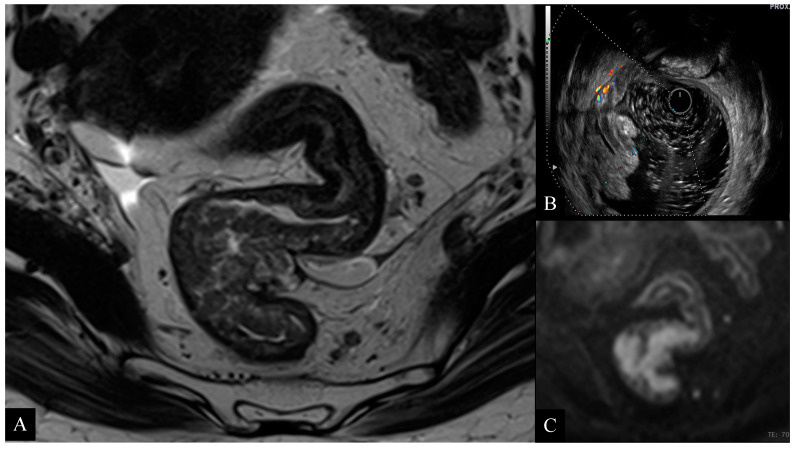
67-year-old woman with high rectal cancer. Axial T2-weighted image (A) obtained with MRI shows a large circumferential tumor infiltrating the muscolaris propria layer and close to the anterior peritoneal reflection. ERUS scan (B) and axial diffusion weighted imaging (DWI) (C) images confirm the presence of a tumor infiltrating the rectal wall with focal invasion to the perirectal fat. Pathology examination confirmed the presence of a T3b tumor.
